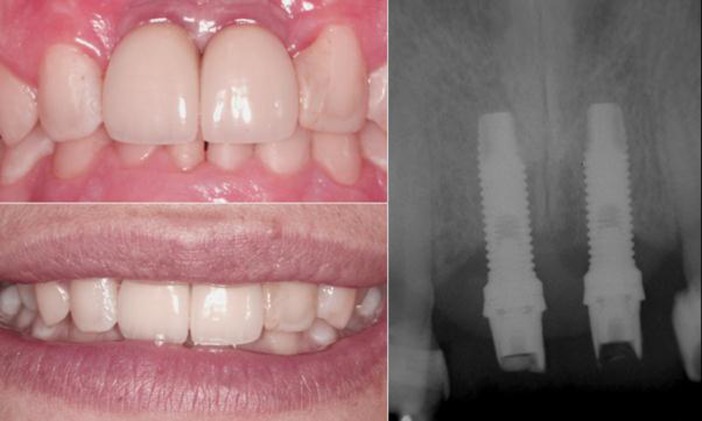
Clinical and radiographic aspect of the final treatment
